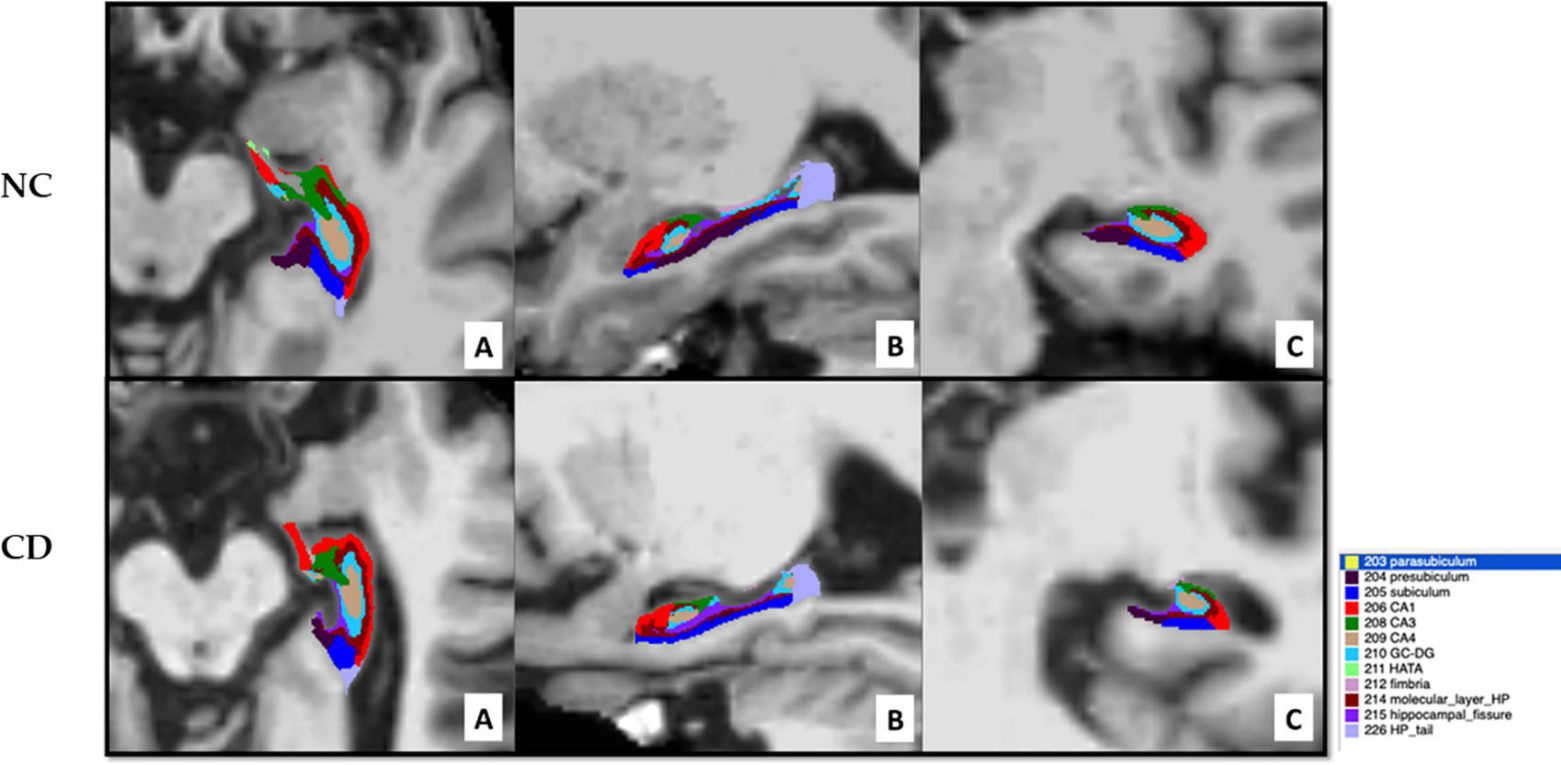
MRI scans of the hippocampus segmentation for a representative NC individual with an MMSE value of 30 (upper panel) and a representative CD patient with an adjMMSE value of 17.9 (lower panel): axial (A), sagittal (B) and coronal (C) view.
